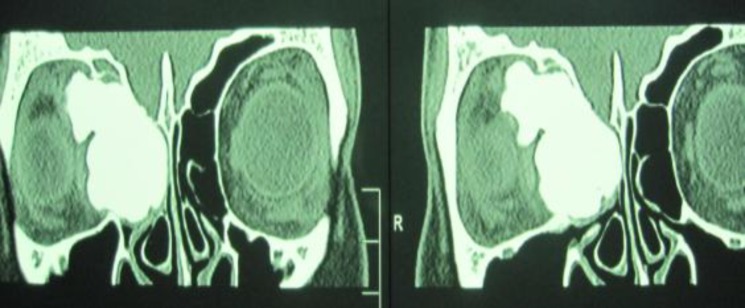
Coronal CT scan showing extension to the orbit
